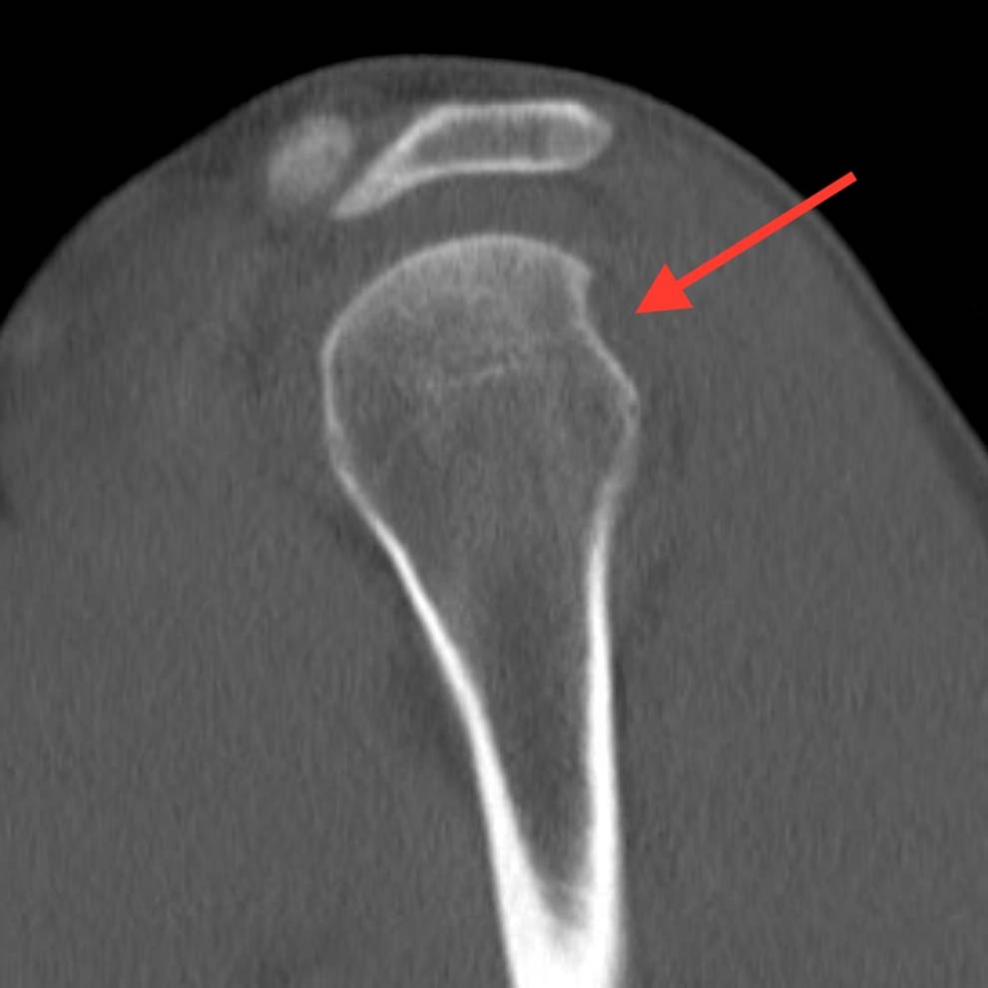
Computed tomography scan of the left shoulder, sagittal cross-sectional image, shows the Hill-Sachs lesion on the posterior humeral head (arrow).
